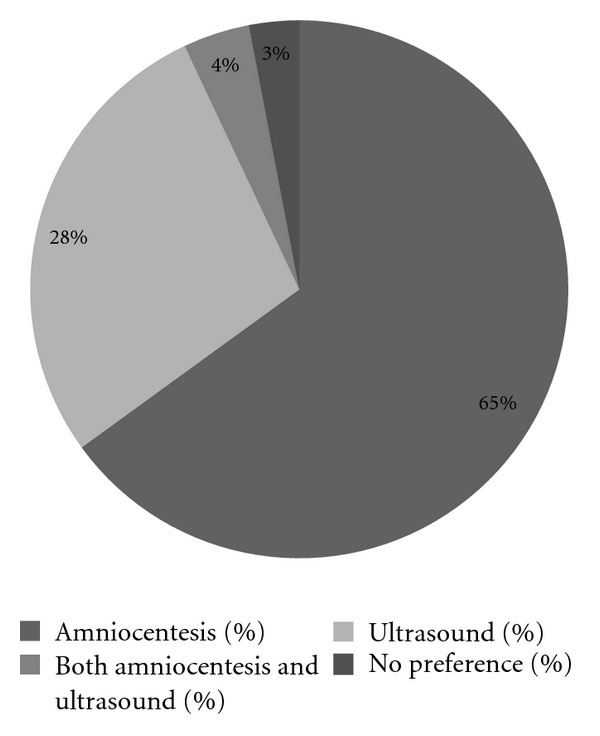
A minority of 28% of the responders were willing to be informed about the fetal gender at the time of the 20-week ultrasound examination, 65% preferred this information to be included in the amniocentesis result, 4% after both tests, and 3% was without preference for either types of test.
